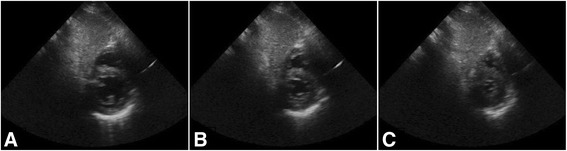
Point-of-care transthoracic echocardiogram subcostal short-axis views. a Short-axis view of the *left* ventricle at the level of the papillary muscles reveals the *left* ventricle initially in diastole, b followed by mid-systole, and c in late systole. Symmetric contraction of the *left* ventricle is appreciated until near obliteration of the *left* ventricle cavity in late systole, which is indicative of a hyperdynamic and under-filled *left* ventricle
